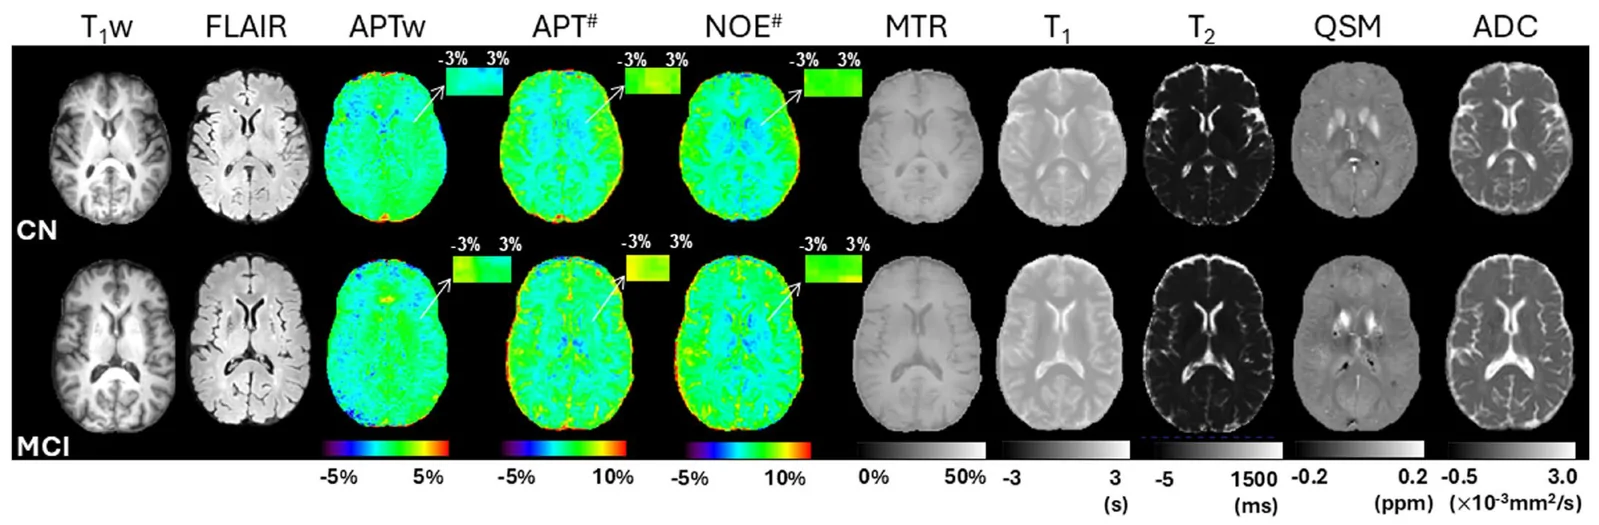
Two examples of multiparametric MR images of CN and MCI/mild dementia participants. CN: 68 years old, Female, MMSE = 27; MCI: 61 years old, Female, MMSE = 19. Higher APTw, APT^#,^ and NOE^#^ signals can be seen in MCI, particularly with small windows (insets).
